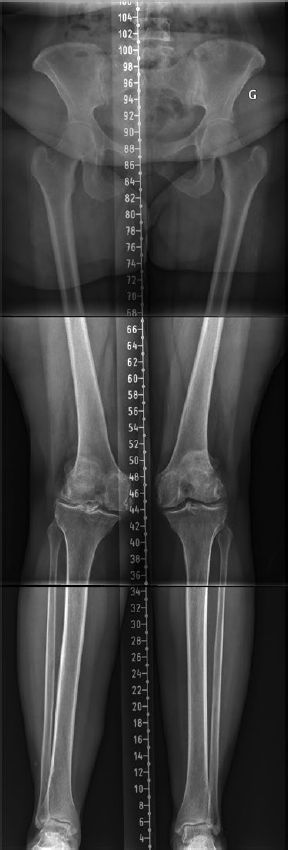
Lower limb long radiographs showing a case with an LDFA of 11° and MPTA of 6°. Reproducing her lower limb alignment with KA technique (unrestricted) would leave her lower limb HKA in 5° of valgus. With rKA, correcting the femur to 5° and the tibia to 2° of varus would results in an HKA of 3° valgus.
